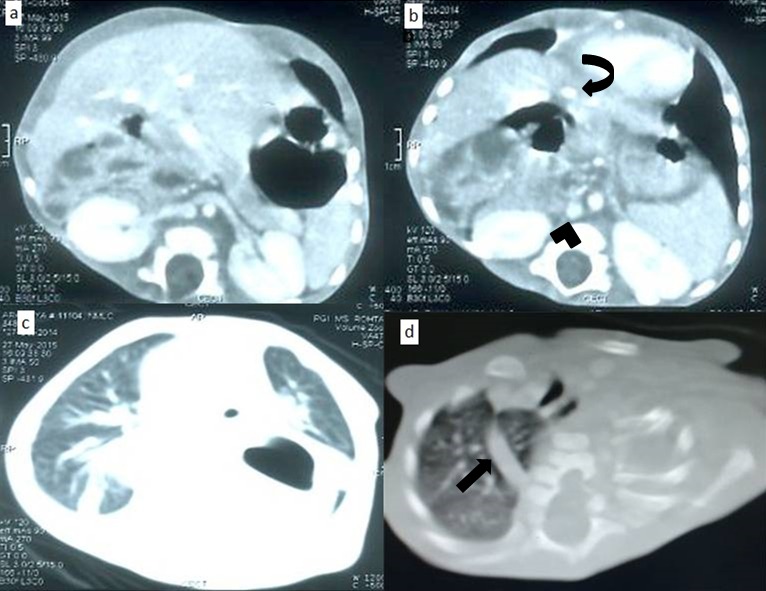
Figure 3: Axial CECT of chest and upper abdomen. (a) Bilateral renal veins are draining into inferior venacava (IVC). Small bowel loops are noted in retroperitoneum in right upper quadrant. (b) All hepatic veins drain into a common channel (curved arrow) draining into right atrium. IVC is seen to continue as azygous vein (arrowhead) (c) Cyst is causing compression of adjacent left lung. (d) CT confirming segmentation, fusion anomalies of lower cervical, upper thoracic vertebrae, left upper rib anomalies. A prominent azygos arch (straight arrow) is evident.
